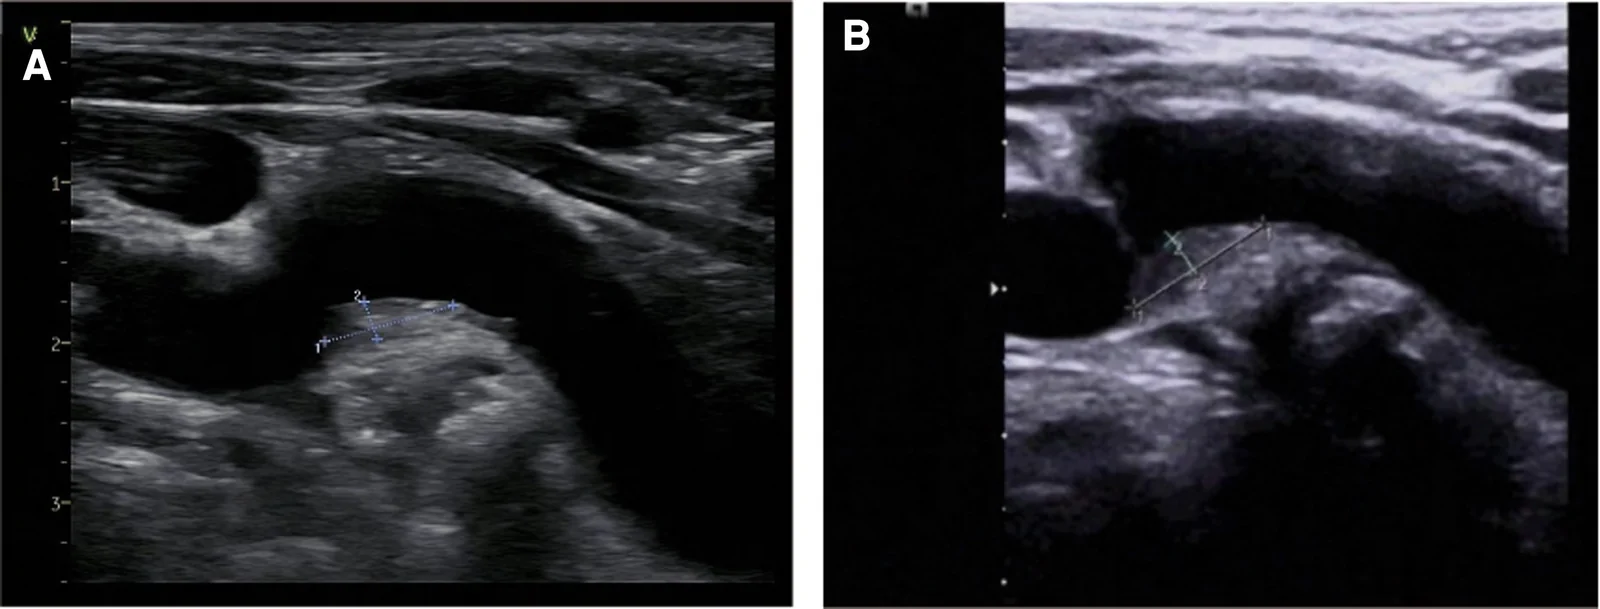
Vascular ultrasound. (*A*) There was an 8.3 × 2.5 mm atheromatous plaque at the right innominate artery bifurcation. (*B*) A persistent 10.0 × 2.9 mm atheromatous plaque was observed at the same site 5 months later. The cross indicates the atheromatous plaque.
